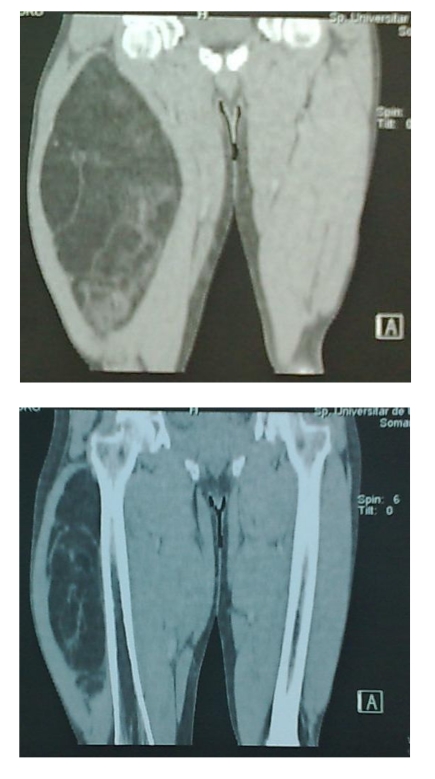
CT scan of the thighs–reconstruction
